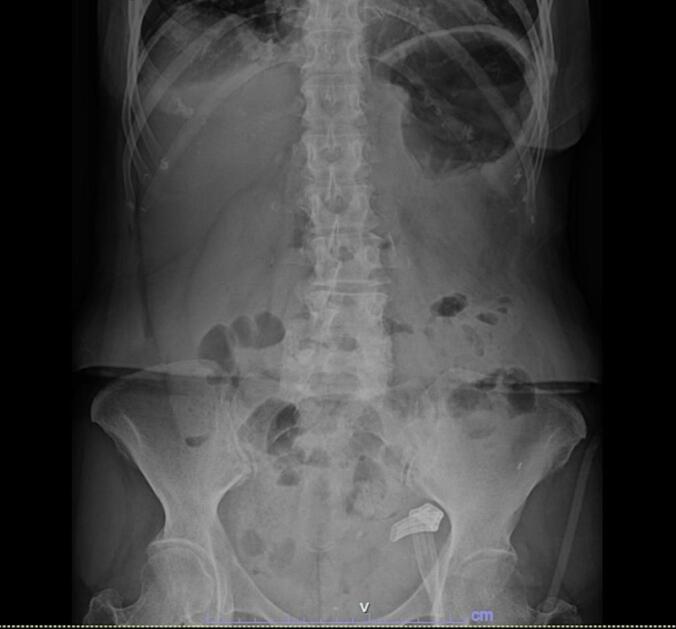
Postoperative radiograph. There is a reduction in intra-abdominal congestion and changes consistent with the colorectal anastomosis.
